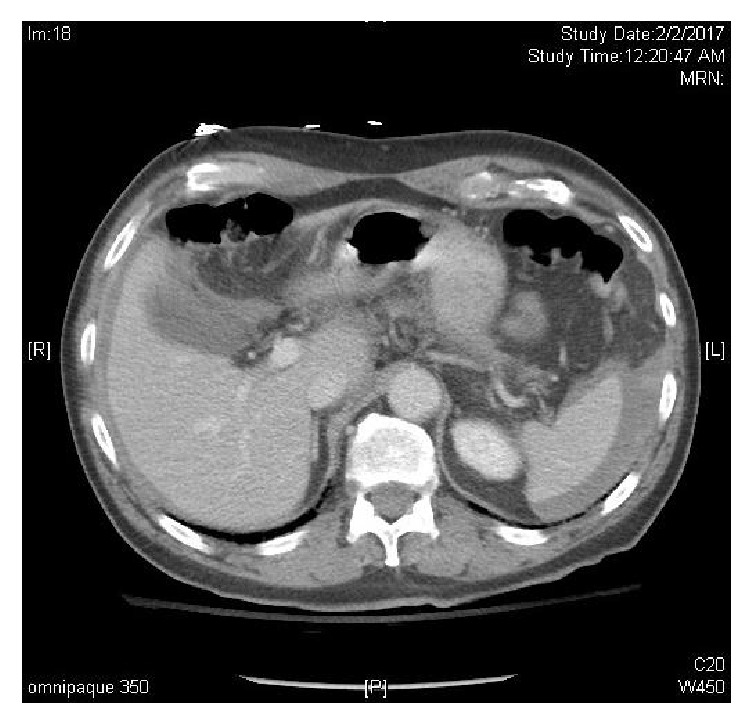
Horizontal CT scan demonstrating hemoperitoneum from iatrogenic spleen injury.
